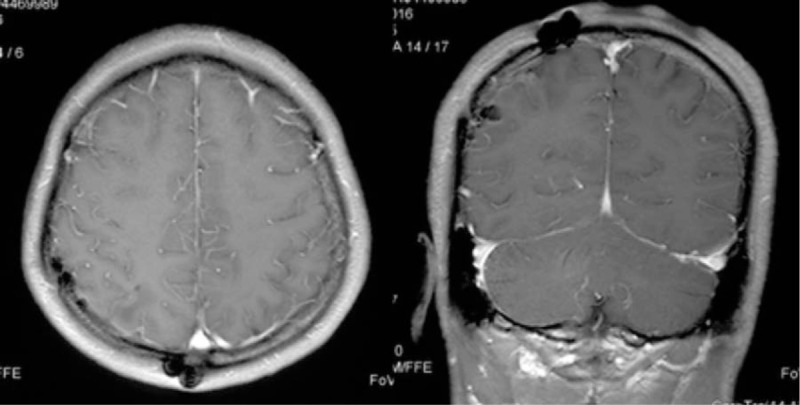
Follow-up MRI in Case 1. Fourteen months after the operation, follow-up MRI showed no tumor recurrence.
